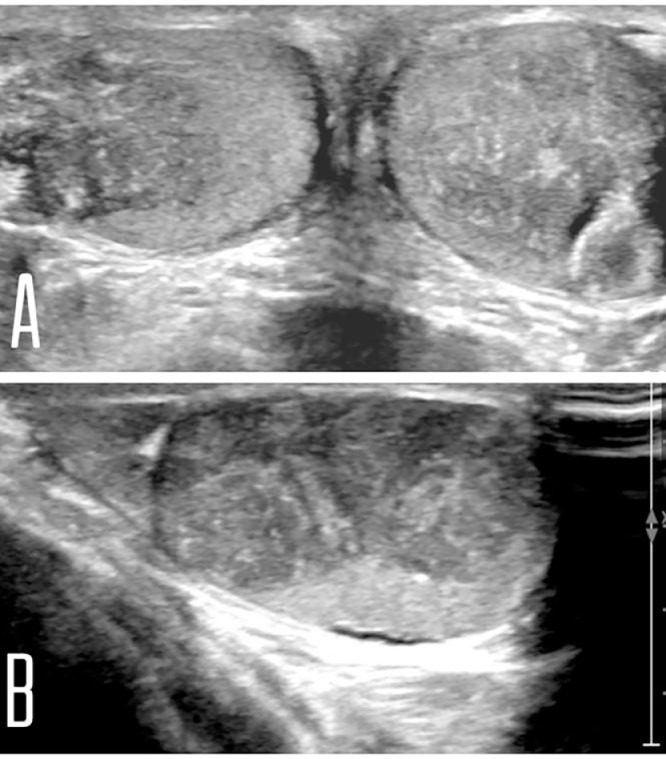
Scrotal ultrasound (A, both testicles, B, right testicle): shows average size testicles with a focal, irregular, eccentric, heterogeneous hypoechoic lesion adjacent to the mediastinum testis in both testicles. No mass effect, narrowing, or distortion is seen traversing through these lesions.
